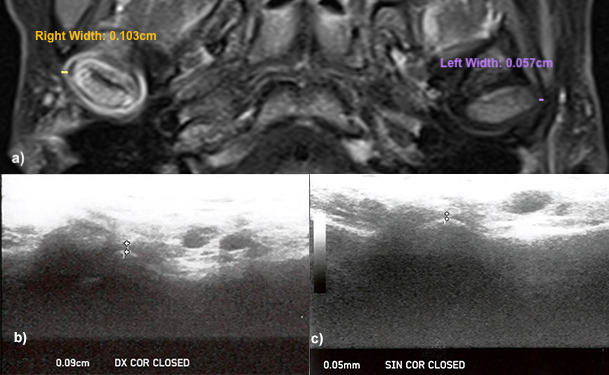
LPAS widths measured in MR and ultrasound images of a Juvenile Idiopathic Arthritis patient with acute involvement of the right temporomandibular joint. (a) Axial contrast enhanced MR image (*T*_1_ weighted TSE). The right temporomandibular joint presents a hyperintense signal (acute inflammation index), with increased LPAS width (0,103 cm). The left temporomandibular joint shows a lower LPAS width (0,057 cm), without acute inflammation. (b, c) Ultrasound images of the right (Dx) and left (SIN) temporomandibular joint. A linear probe in Coronal (COR) direction was used and the patient was in a closed-mouth position. Ultrasound images show a LPAS width of 0,09 cm in the right temporomandibular joint and a LPAS width of 0,05 cm in the left temporomandibular joint. LPAS, lateral periarticularspace; TSE, turbo spin echo.
